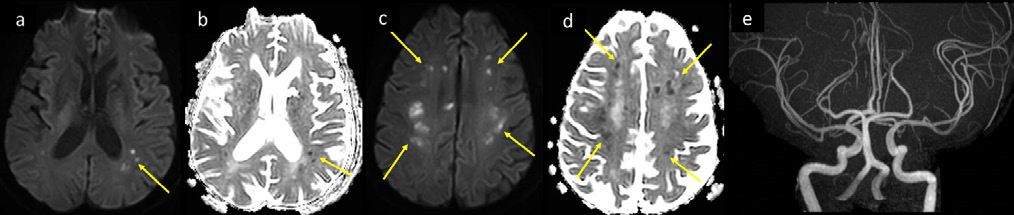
CNS vasculitis like changes in a 56-year-old male patient with COVID-19 severe ARDS. He was intubated with stabilization of pulmonary function and oxygen saturation, blood pressure was controlled, and coagulation panel was normalized. He remained drowsy and non-arousable. MRI axial DWI and ADC, (a, b) at the ventricular level, and (c, d) at supraventricular level showing left frontoparietal periventricular and multilobar bilateral deep subcortical recent lacunar infracts with diffusion restriction with punctate and nodular foci of bright DWI and corresponding low signal on ADC (arrows in a–d). The intracranial MRA (f) showed normal appearance of arteries. ADC, apparent diffusion coefficient; ARDS, acute respiratory distress syndrome; CNS, central nervous system; DWI, diffusion-weighted image; MRA,MR angiography.
